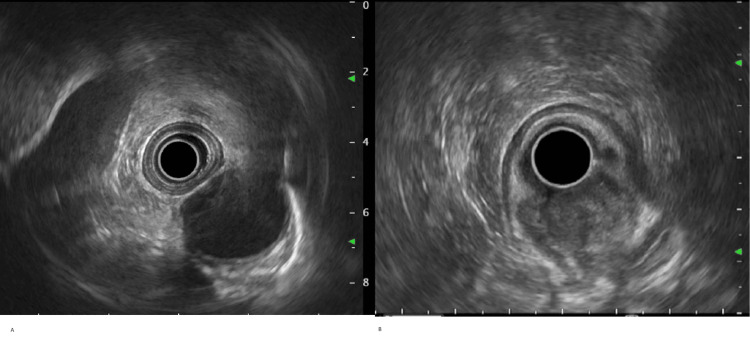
Endoscopic ultrasound (A) There was no sign of significant endosonographic abnormality in the proximal and middle esophagus. (B) A hypoechoic oval mass was identified endosonographically, in the gastroesophageal junction, extending to cardia of the stomach. This was staged T1sm N0 Mx by endosonographic criteria. There was sonographic evidence suggesting invasion into the submucosa (layer 3). Images reproduced with patient permission.
